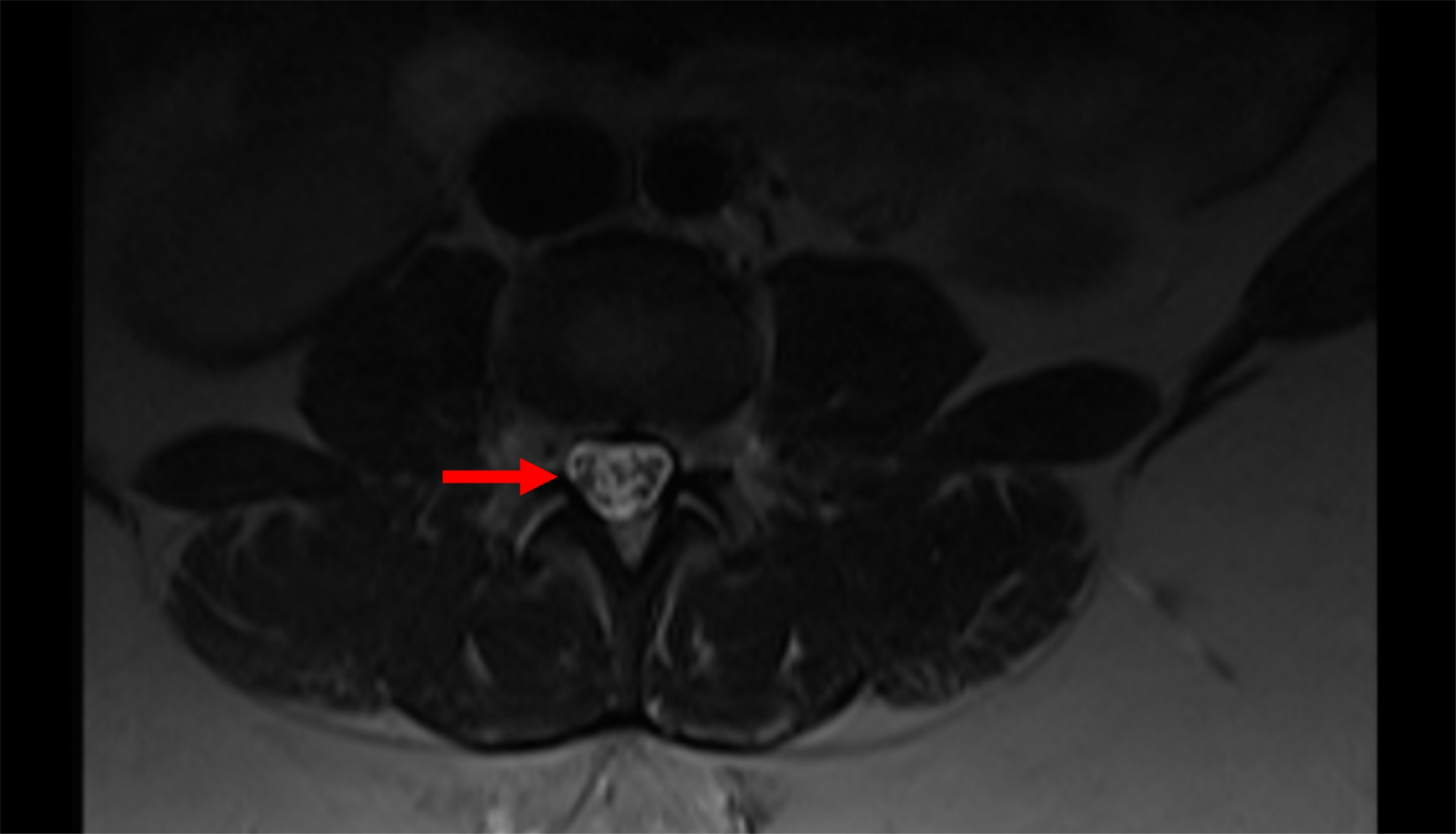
Axial MRI Image Axial normal image 2 cm below the lesion, showing the normal position of the nerve roots.
